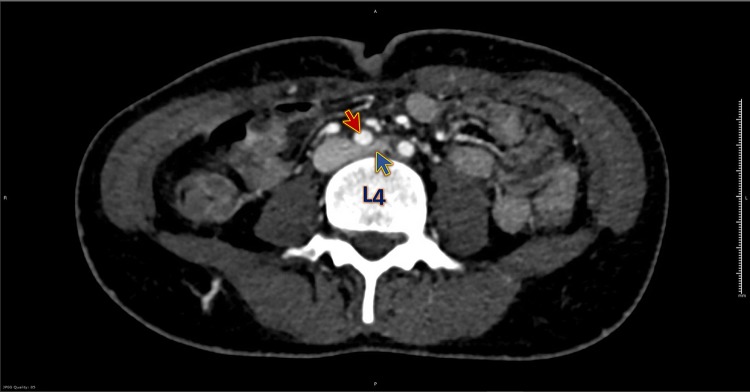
CT venogram axial view shows compression of the left iliac vein (blue arrow) by the right iliac artery (red arrow) at the level of the 4th lumbar vertebra (L4).
